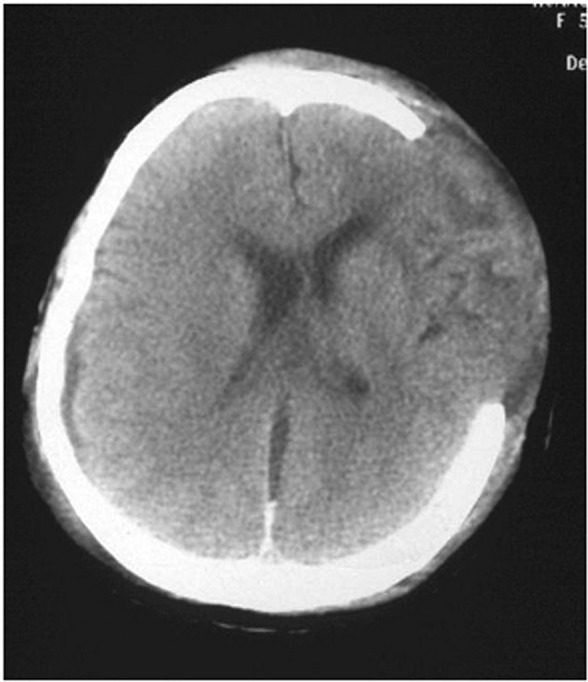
Axial computed tomography scan with a bone window demonstrates a marked bulging of the brain tissue, which caused the mid-line and the ventricular system to shift to the left.
